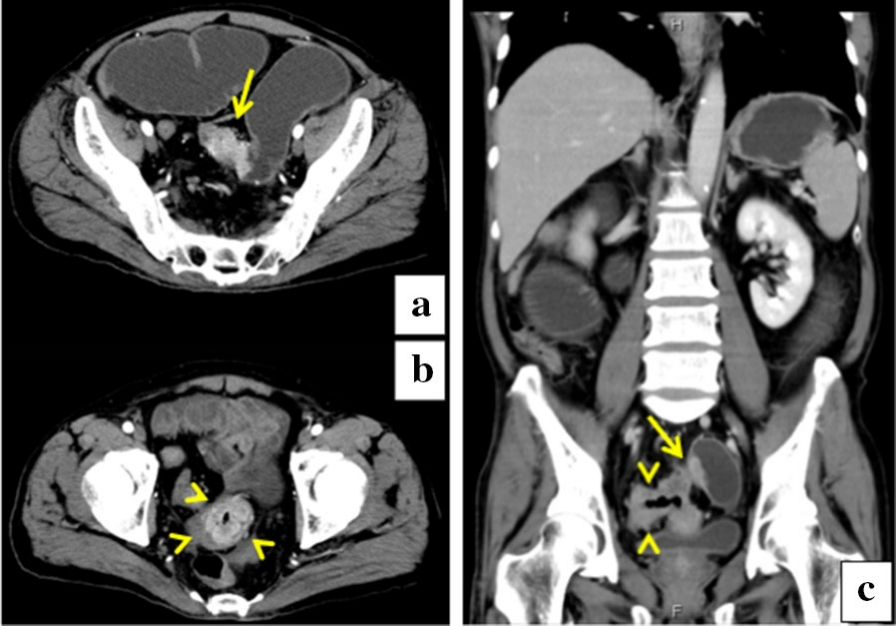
a–c CT revealed a 6.5-cm-sized sigmoid colon cancer. Tumor invasion was extended to the ileum and caused the small intestine ileus. The arrowhead shows sigmoidal tumor, and the arrow shows ileal invasion
